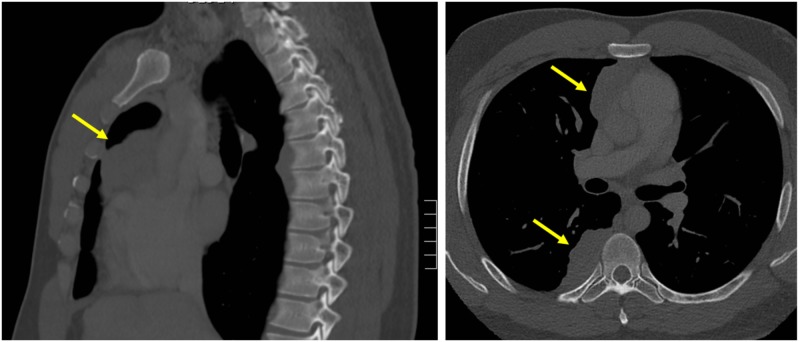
CT Chest demonstrating thymoma in anterior mediastinal space with pleural metastasis
